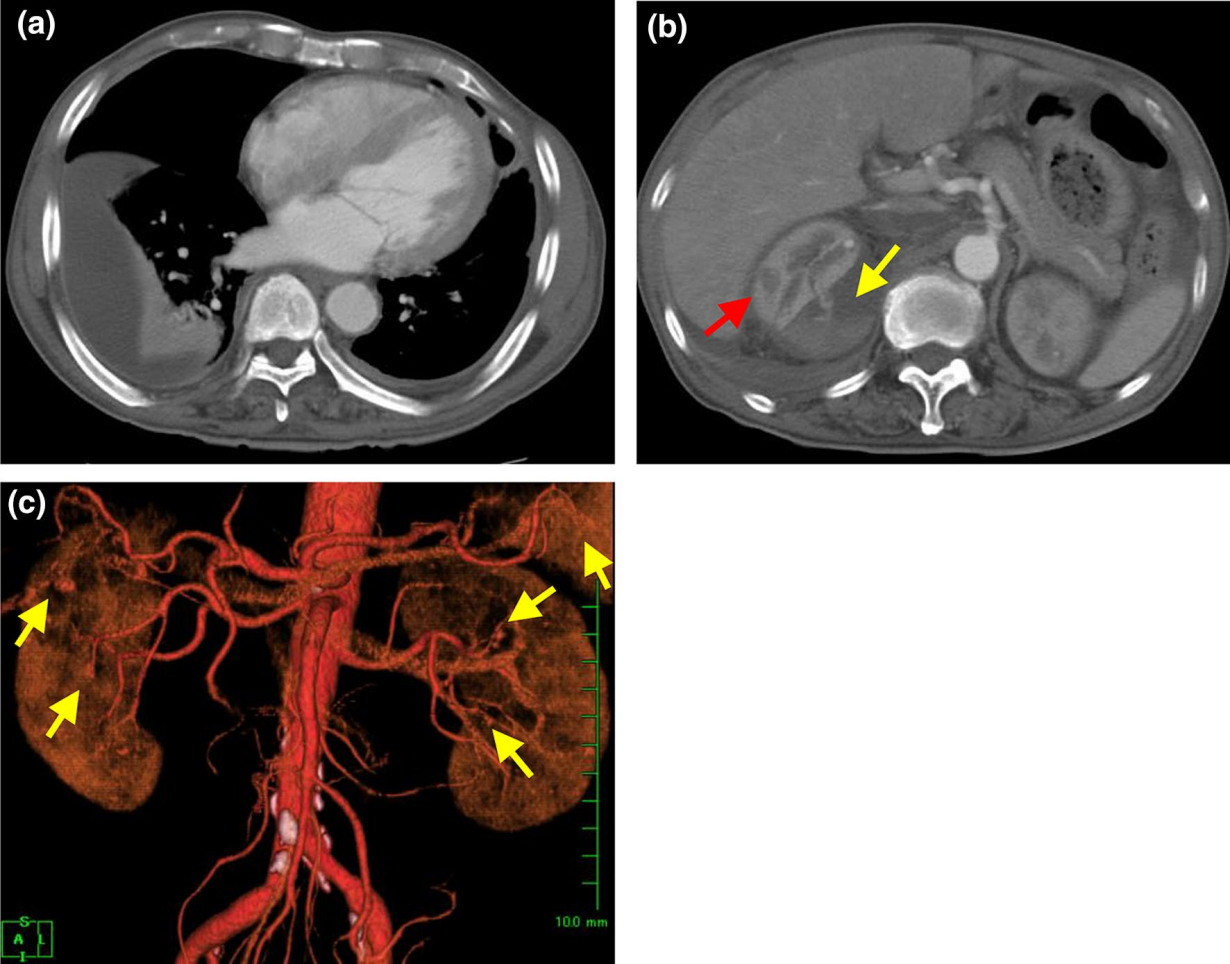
Contrast-enhanced computed tomography. a Bilateral pleural effusion was apparent. b CT scan image showing a right perirenal hematoma with extravasation of contrast media (yellow arrow) and a small renal infarction (red arrow). c CT angiography showing multiple small aneurysmal dilatations in the intrarenal branches of the bilateral renal arteries and in the intrasplenic branches of the splenic artery (yellow arrow)
